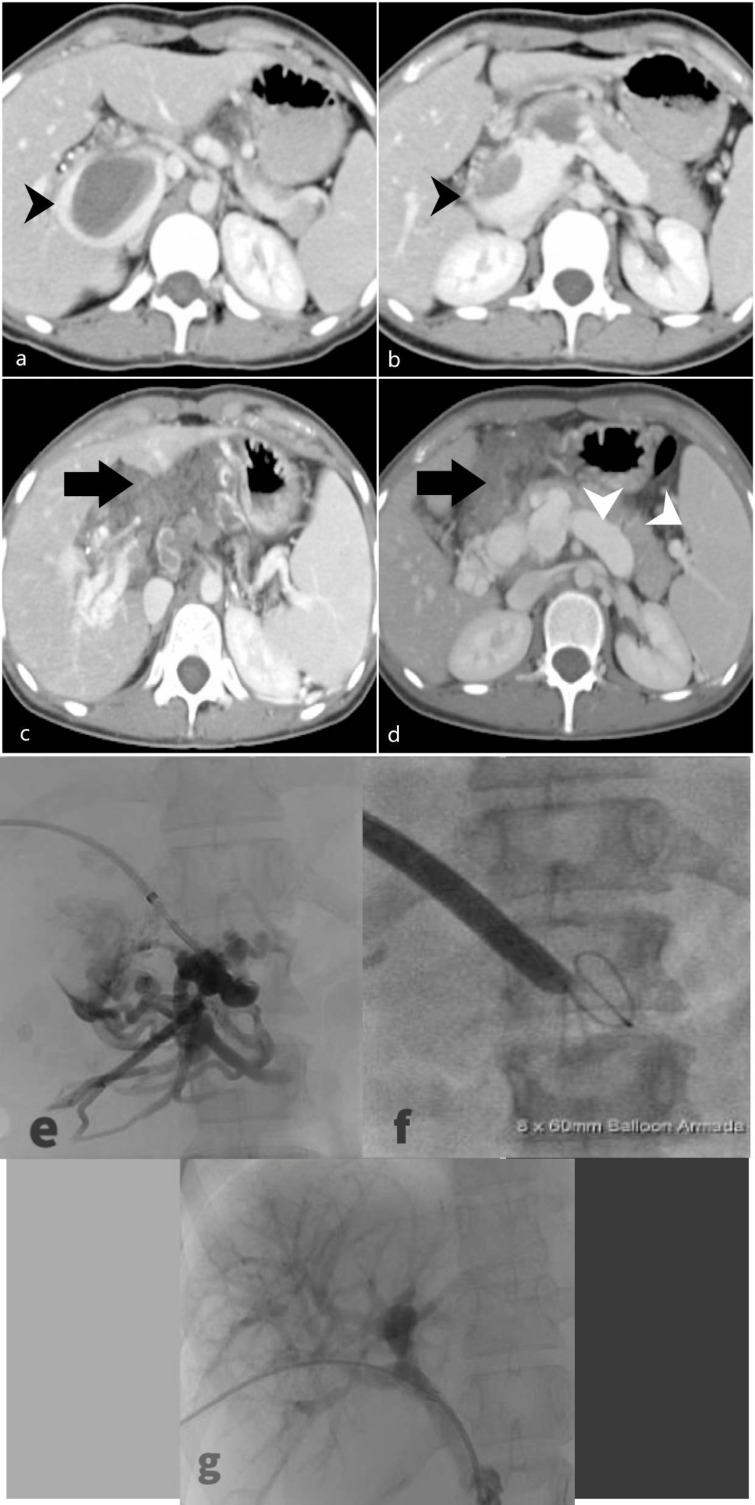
(a, b) Axial CT images in the portal venous phase showed a 4 cm aneurysmal dilatation of the intrahepatic portion of main portal vein. A large filling defect of varying densities seen within, which likely represent different ages of a thrombus (black arrowhead). (c, d) Follow-up CT scan 2 years later showed chronic thrombosis of the main portal vein with cavernous transformation (black arrow) with an apparent acute thrombus seen within the collateral vessels. The distal superior mesenteric vein is aneurysmal and splenic vein is dilated with progressive splenomegaly, related to portal hypertension (white arrowhead). (e) Portal venogram demonstrating occlusion of the extrahepatic main portal vein. (f) Portal vein access balloon dilatation of the vein was performed. (g) Post-venoplasty venogram showed adequate flow in the portal vein.
